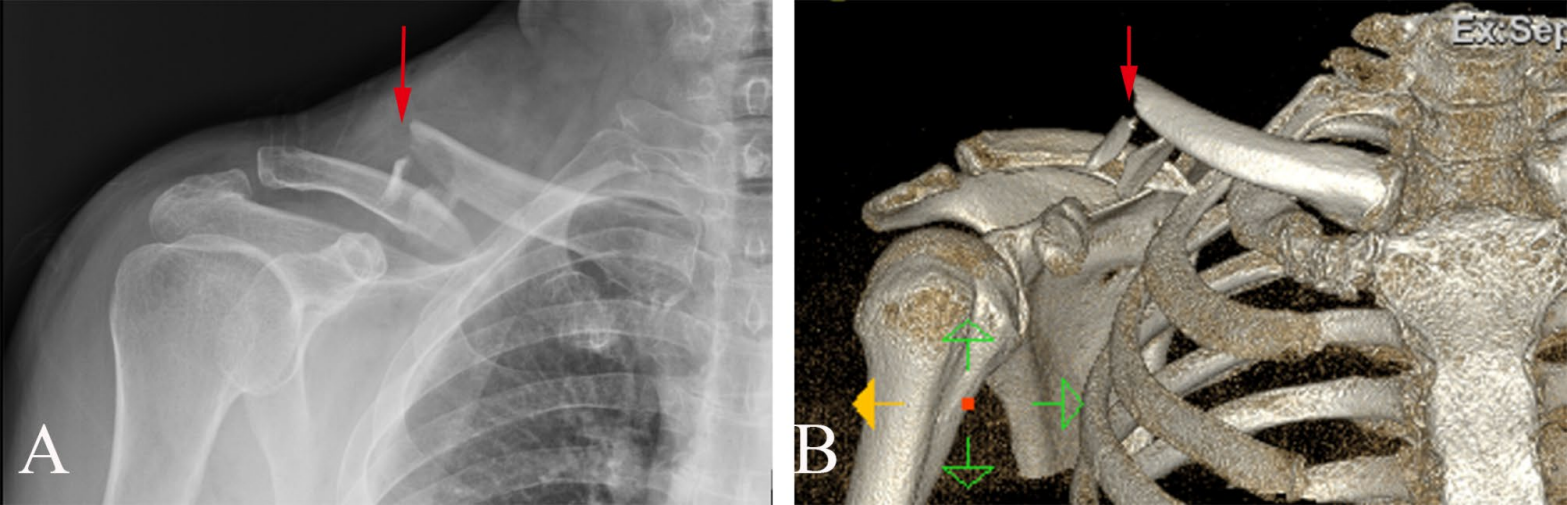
Preoperative AP radiograph and 3D CT reconstruction of a clavicular fracture A: AP radiograph. B: 3D CT reconstruction. The red arrow indicates the fracture site and comminuted fragments
